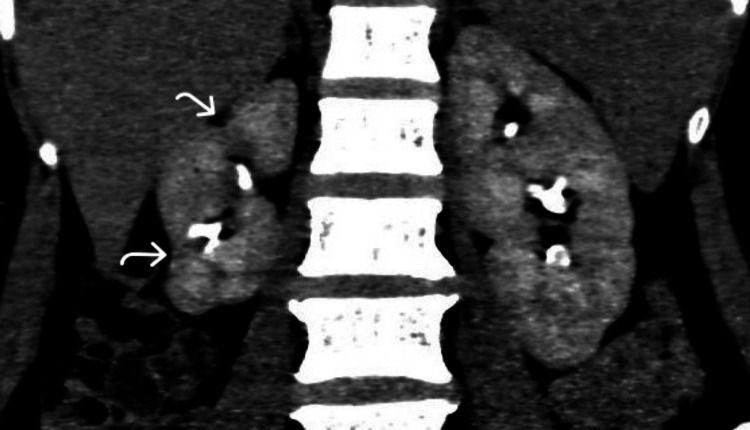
Chronic pyelonephritis. A 55-year-old woman with right loin pain was diagnosed with chronic pyelonephritis. Coronal reformatted image of delayed phase CT urogram demonstrates a shrunken right kidney with irregular contour and focal cortical scars (curved arrows) in the polar regions. These images are from the hospital and have not been published elsewhere. CT: computed tomography
